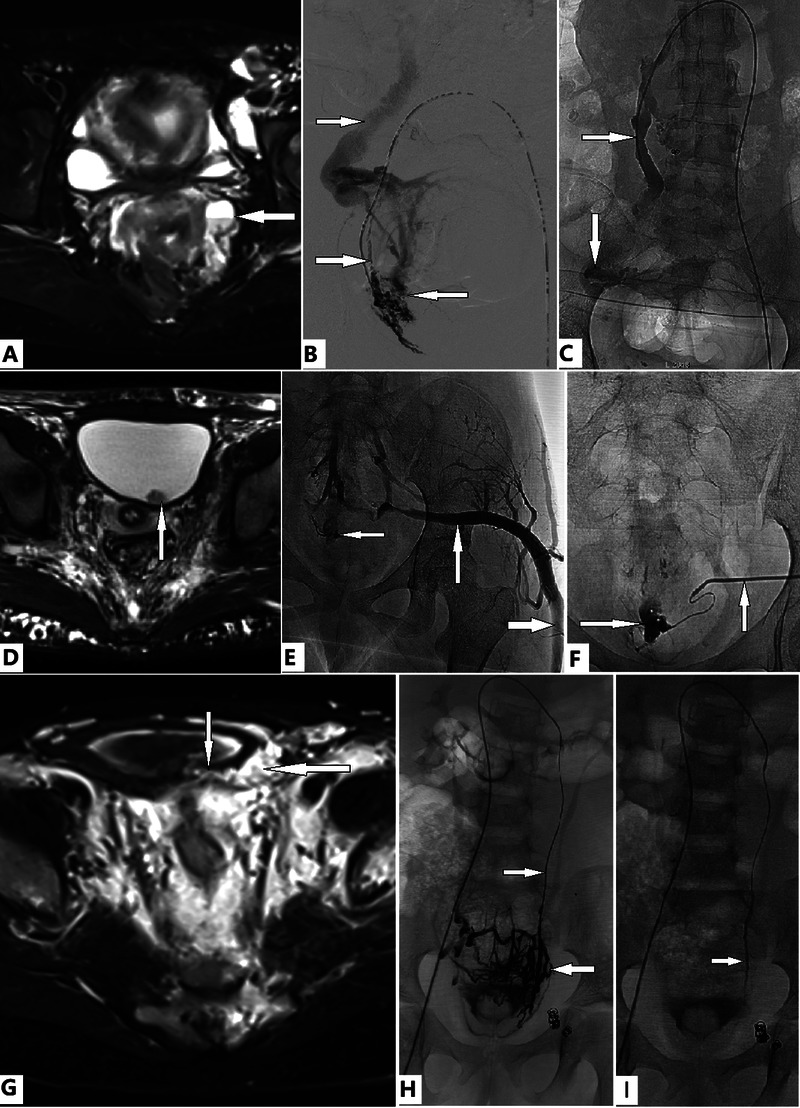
Evaluation and transcatheter treatment of genitourinary (GU) involvement in female patients with Klippel–Trénaunay syndrome (KTS). (A–C) A 38-year old woman with KTS presented with dysmenorrhea and severe anemia due to menorrhagia for years, which required multiple medications and repeated blood transfusions. T2 Magnetic resonance imaging (T2 MRI) reveals the ovary (A, arrow) and uterine venous malformation (VM). She is in the prone position. Transcatheter uterine venography reveals VM of the uterus and ovary (B, leftward arrow), dual draining into the uterine veins (B, lower rightward arrow), and the left ovarian vein (LOV), which is significantly dilated and incompetent (B, upper rightward arrow). The uterine VM was managed with transcatheter ethanol and foam polidocanol. Treatment of the ovarian VM (C, downward arrow) through the LOV (C, rightward arrow) was performed in the same fashion. The patient's anemia and menorrhagia resolved completely. Complete relief from dysmenorrhea was obtained after two sessions of treatment. (D–F) A 14-year old girl with bilateral KTS presented with severe anemia due to persistent gross hematuria. Bladder bleeding is identified on magnetic resonance imaging (D, arrow). The active bleeding site is identified by venography (E, leftward arrow). The marginal superior gluteal venous channel (E, rightward and upward arrows) is large and connected to the bleeding VM of the bladder (E, leftward arrow; F, rightward arrow). Transmicrocatheter management through the marginal superior gluteal venous channel (F, upward arrow) is performed in the same fashion, as described previously. Anemia and hematuria completely resolved during follow-up. (G–I) A 5-year old girl with KTS presented with moderate anemia due to intermittent gross hematuria. T2 MRI revealed that the bladder wall (G, downward arrow) was infiltrated by a VM (G, leftward arrow). She had previously undergone transcatheter coil embolization and sclerotherapy of the left IIV; however, gross hematuria persisted. Dilation of the LOV (H and I, rightward arrows) is detected on imaging. Transmicrocatheter management through the left ovarian vein (LOV) was performed by using ethanol. The venous plexuses of the ovary and bladder are significantly intercommunicated (H, leftward arrow) and ablated with ethanol (I). The anemia and hematuria resolved completely after treatment.
